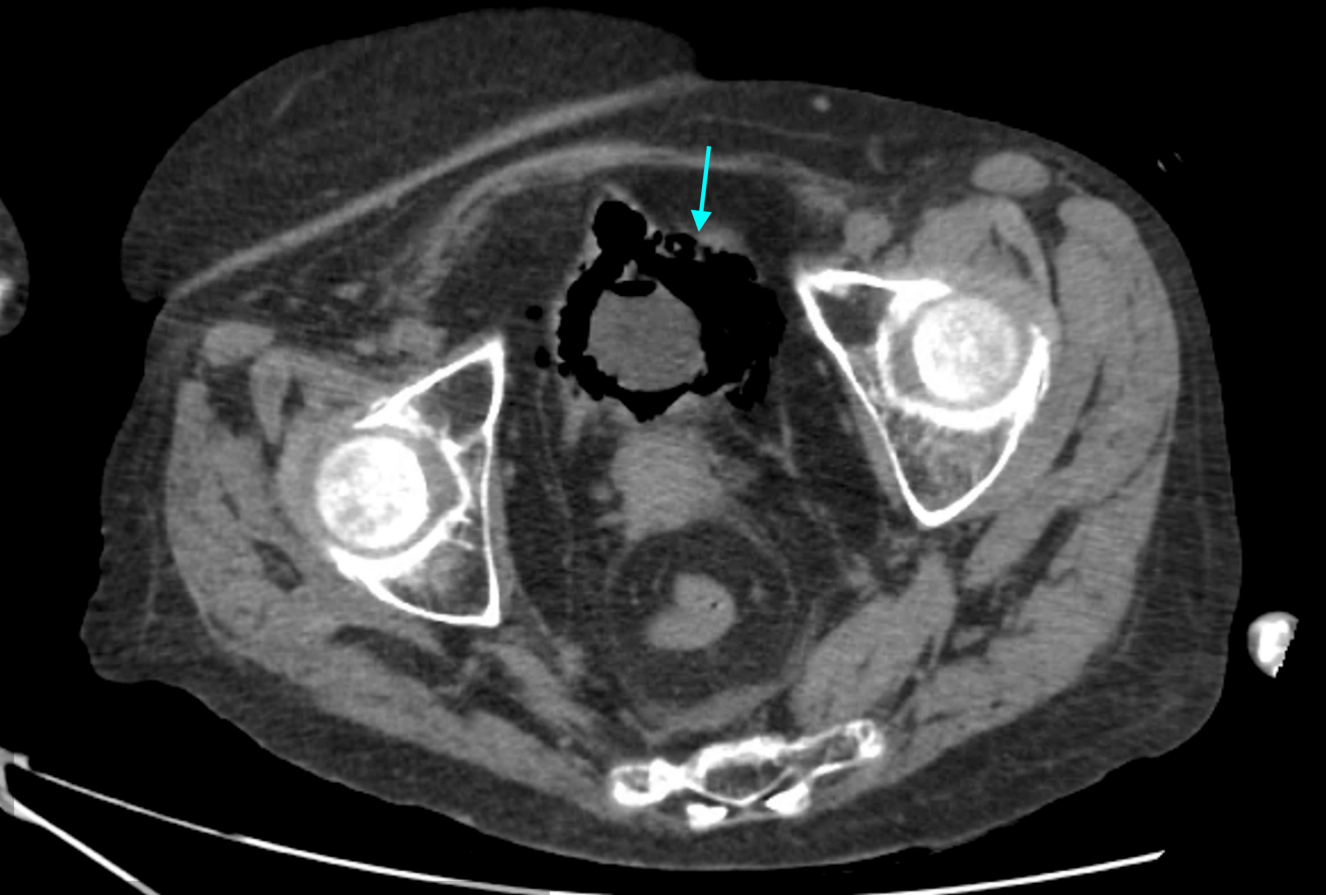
Axial computed tomography image showing circumferential intramural gas within the bladder wall and surrounding perivesical emphysema, characteristic of emphysematous cystitis
